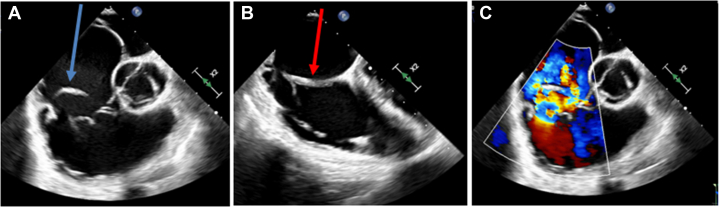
Transesophageal Echocardiography Focused on the Tricuspid Valve (A) Aspect of posterior tricuspid leaflet flail (blue arrow). (B) Aspect of “ghost material” (red arrow) looking like “aberrant” chordae attached from the lateral wall of the right atrium to the tricuspid valve. (C) Massive tricuspid regurgitation with eccentric jet.
